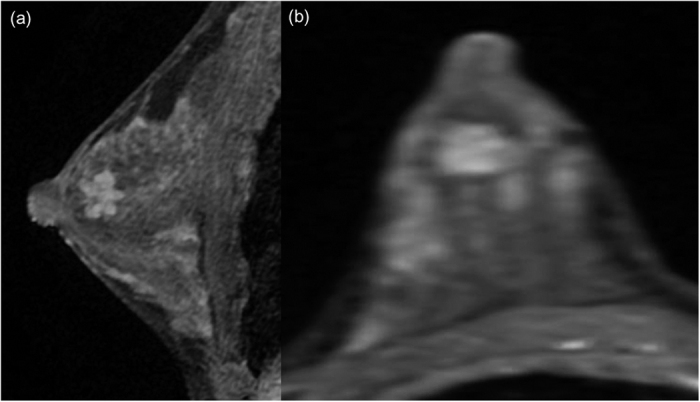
A fibroadenoma in the right breast of a 26-year-old woman. (a) Sagittal contrast-enhanced non-subtracted T1-weighted MR image with fat suppression shows a 1.5 cm circumscribed mass. Note the mass located within the glandular tissue. (b) Subtracted axial reformatted image.
